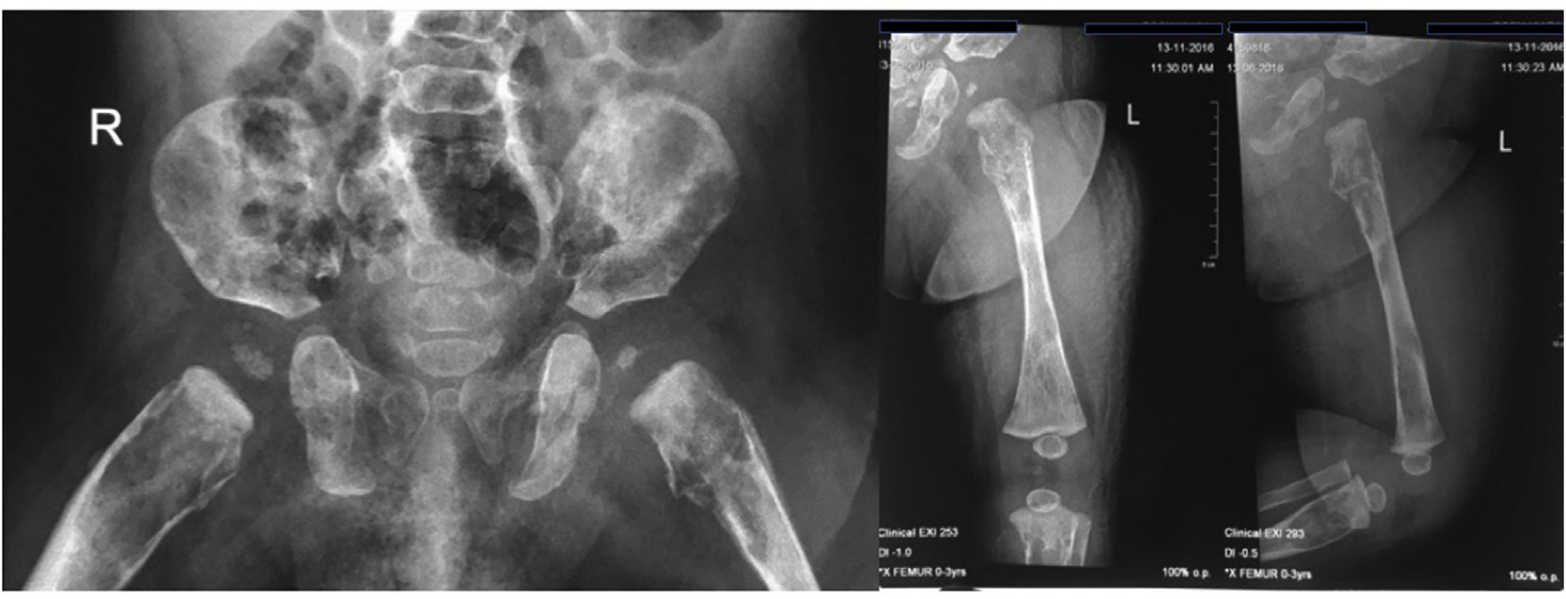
Initial X-rays of pelvic and left femur showed multiple lytic lesions and thinning of bone cortices.
